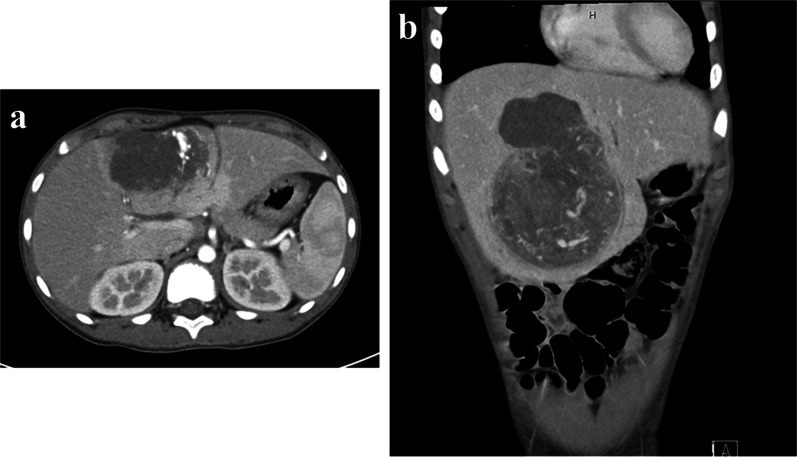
CT findings. CT shows a 7.7 × 8.8 × 12.0-cm tumor located mainly in S4 and S5 of the liver (a). It was well-circumscribed and showed gradual weak enhancement in both the arterial and portal phases (b)
